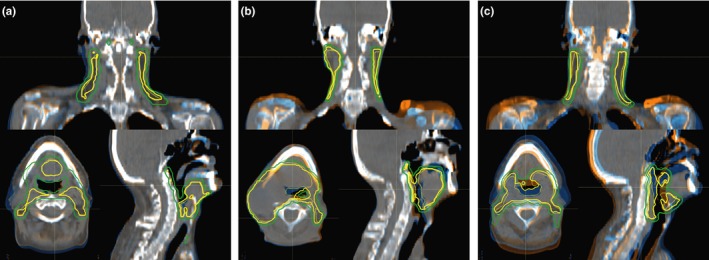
Examples for differently rated matching between CT
